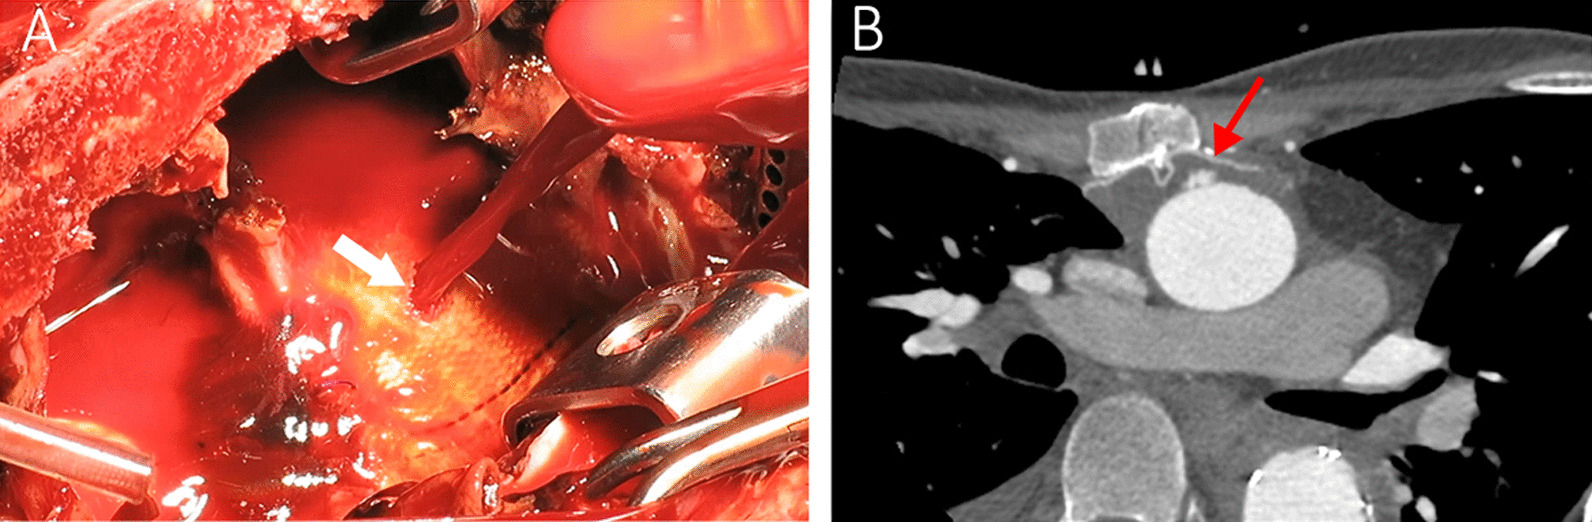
A The erosion hole on the Dacron graft and the outburst of blood shown by a white arrow. B The CTA performed three months after the repair. Note the Gore-tex patch placed between the sternum and the aortic graft
